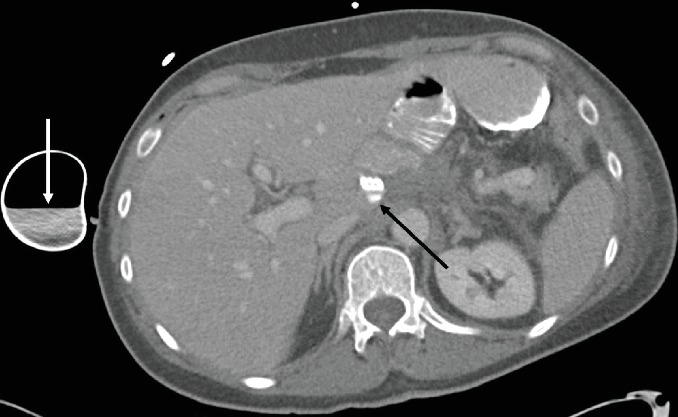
Axial postcontrast CT performed in the postoperative period showed leaked contrast concentrated at the tip of the surgical drain (black arrow). Contrast level is also present within Jackson-Pratt drain reservoir (white arrow).
